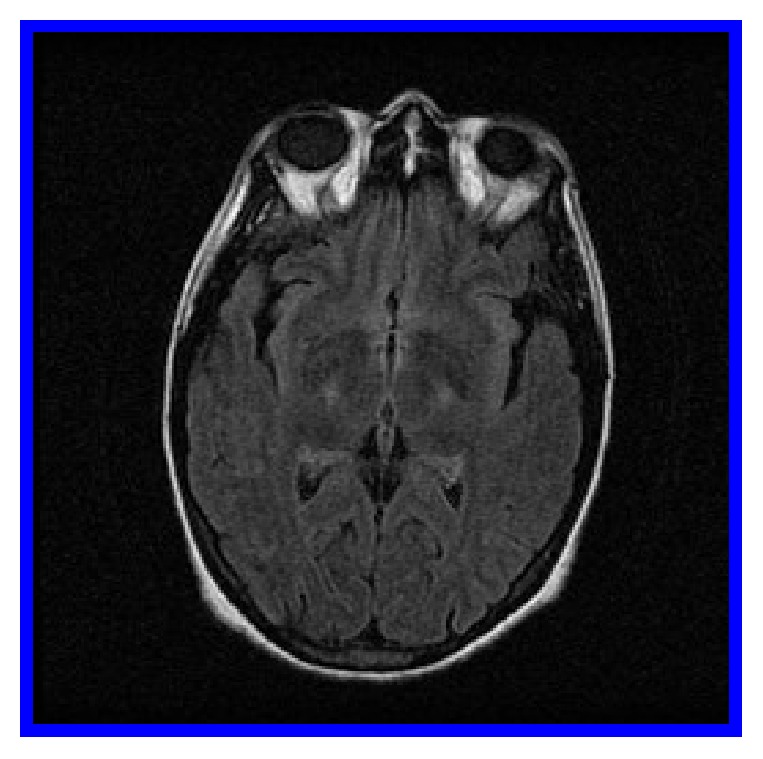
Axial brain MRI, FLAIR sequence, showing bilateral hyperintensities of the basal ganglia prior to transplantation.
